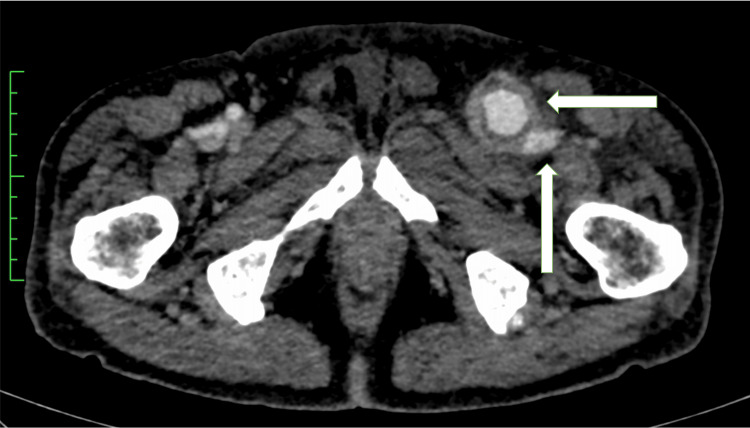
The preoperative CECT image On the preoperative CECT image, the left CFA shows an enlargement of 3.1×3.4 cm in diameter, marked wall thickening, and enlarged surrounding lymph nodes (⇦), while the left SFV is compressed (⇧). CECT - contrast-enhanced computed tomography; CFA - common femoral artery; SFV - superficial femoral vein
